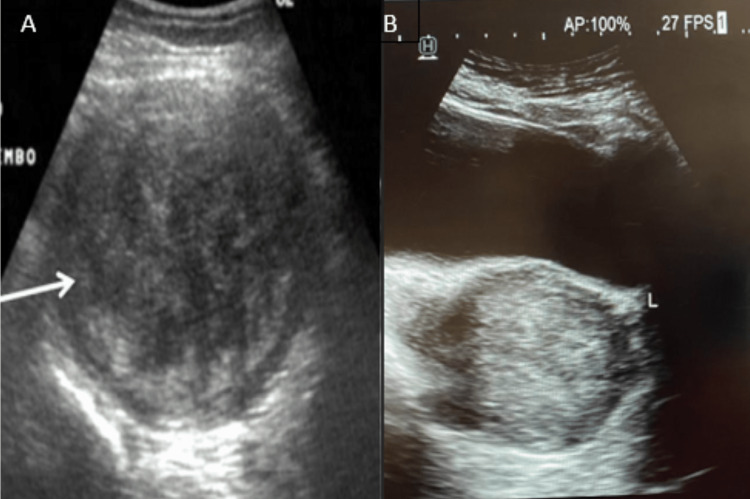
Shows USG comparing the size of fibroid pre-embolization (8cmx7cm) (Figure A) and post-embolization (5cmx4cm) (Figure B) USG: Ultrasonography
